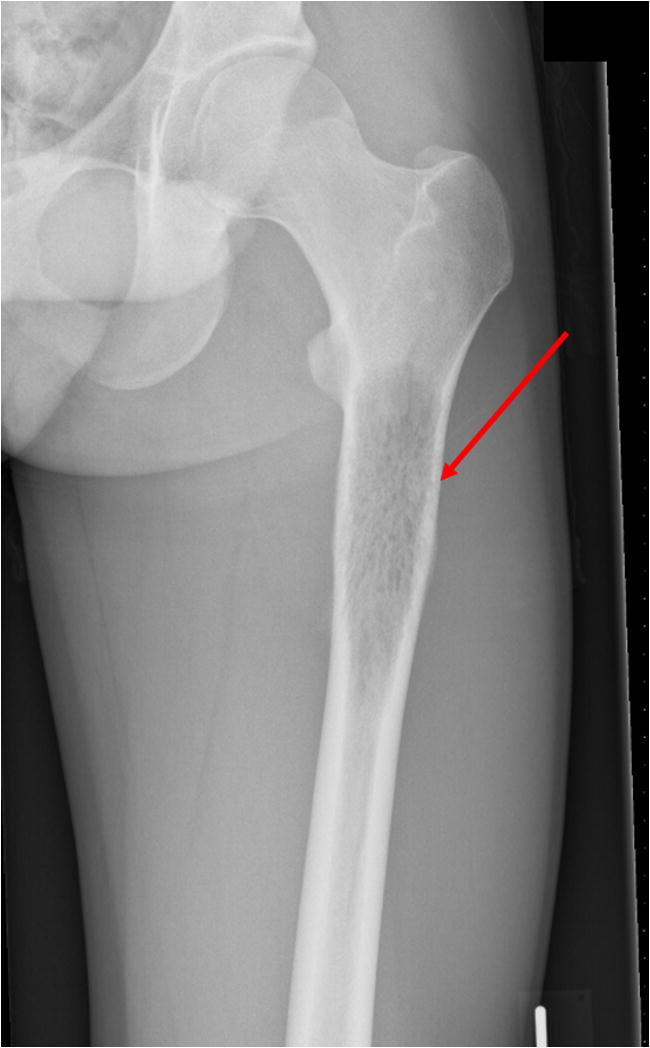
X-ray of the left femur demonstrating destruction of left femoral cortex due to bone metastasis
